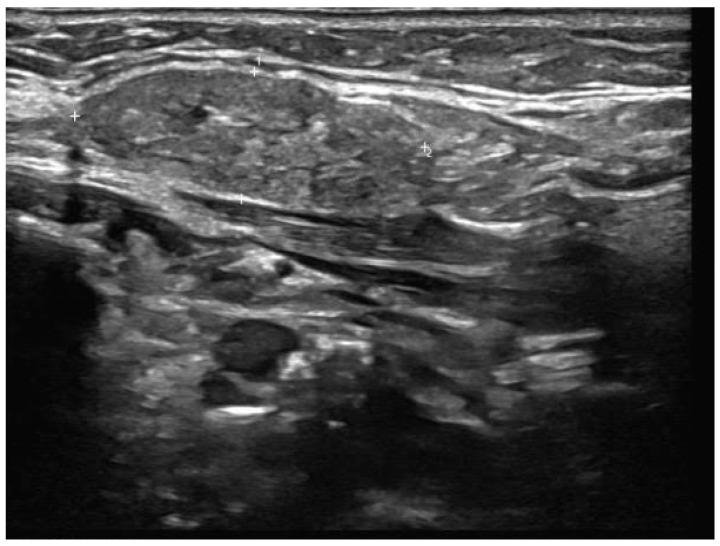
Patient Y—Follow-up ultrasound scan of affected SMG. Complete spontaneous resolution of previously identified lesion on follow-up imaging. The "+" markers represent measurement callipers.
